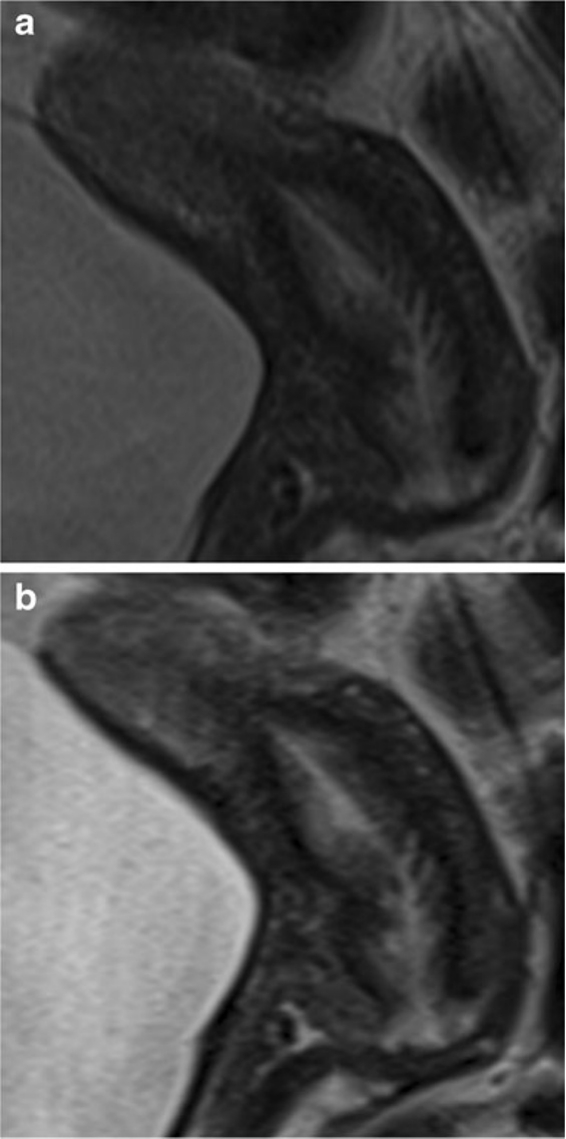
MRI of the normal anatomy of the cervix in a pre-menopausal 35-year-old patient with endometriosis. a Sagittal T2-weighted BLADE sequence. b Sagittal T2-weighted conventional TSE sequence. The cervical mucosa and stroma are more sharpely delineated on the BLADE image (a); however, SNR and CNR are higher on the conventional TSE image in b
